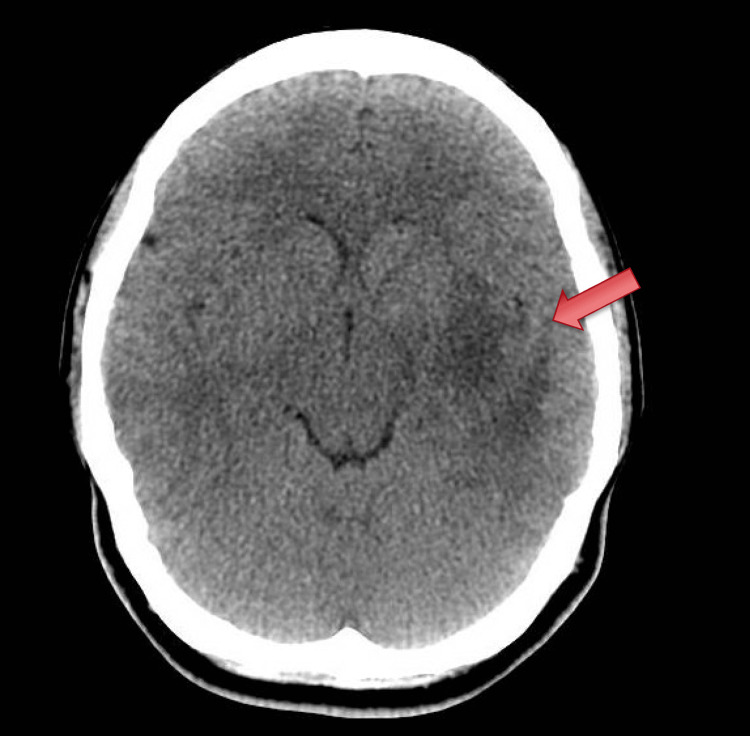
Non-contrast CT of the head revealing a large area of vasogenic edema in the left frontal and temporal lobes. CT, computed tomography
